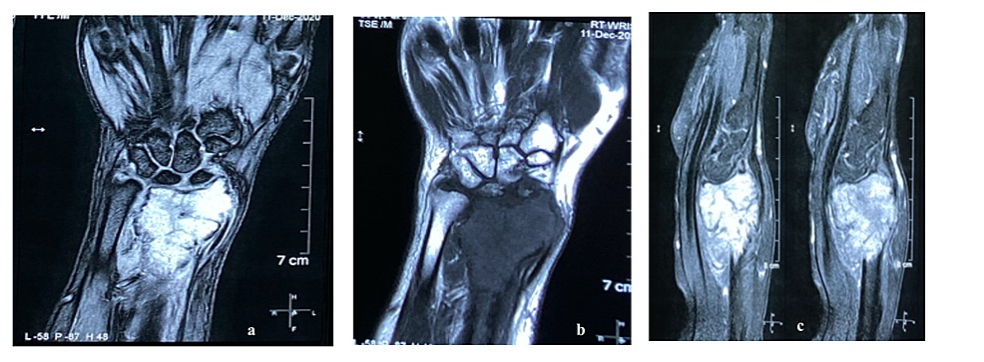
(a & c) MRI T2 image showing lesion at distal radius and extra compartmental involvement, and (b) MRI T1 image showing the extent of the lesion.
